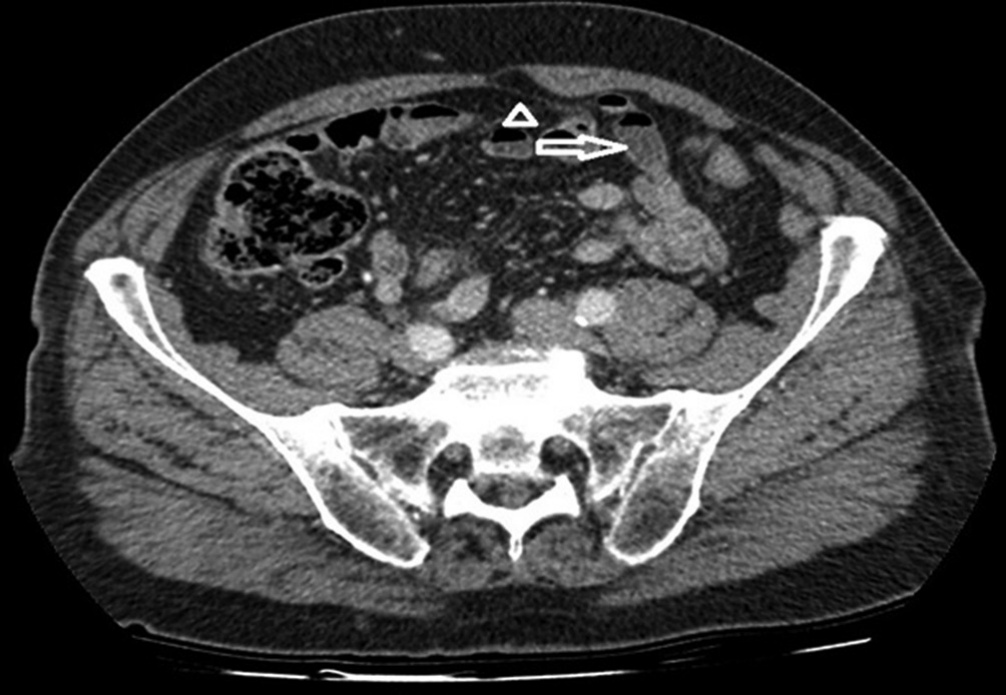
Another contrast-enhanced portal venous phase axial CT image again shows the split sheath (arrowhead) and a bowel loop entering the defect (arrow).
